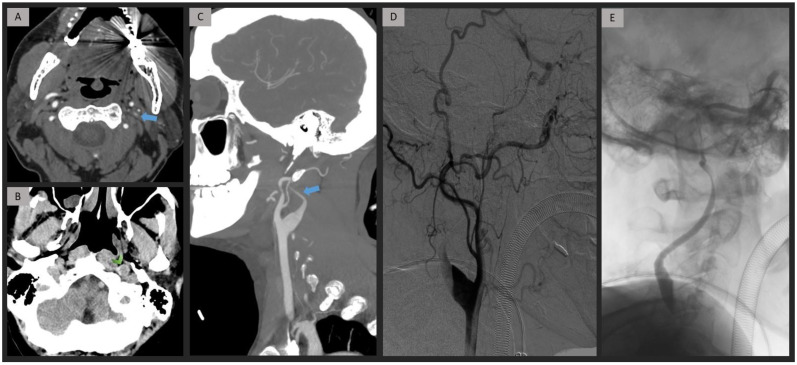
Patient presenting with left extracranial ICA occlusion caused by dissection. Axial and Sagittal-MIP CTA images (A,C) demonstrate a progressive contrast decay and stenosis of the cervical ICA, associated with an arterial widening (blue arrow). NCCT (B) reveals a wall hematoma in the left ICA near the skull base (green arrowhead). DSA (D) and fluoroscopy (E) confirm the diagnosis of cervical ICA dissection. ICA = internal carotid artery; NCCT = non-contrast computed tomography; MIP = maximum intensity projection; CTA = computed tomography angiography; DSA = digital subtraction angiography.
